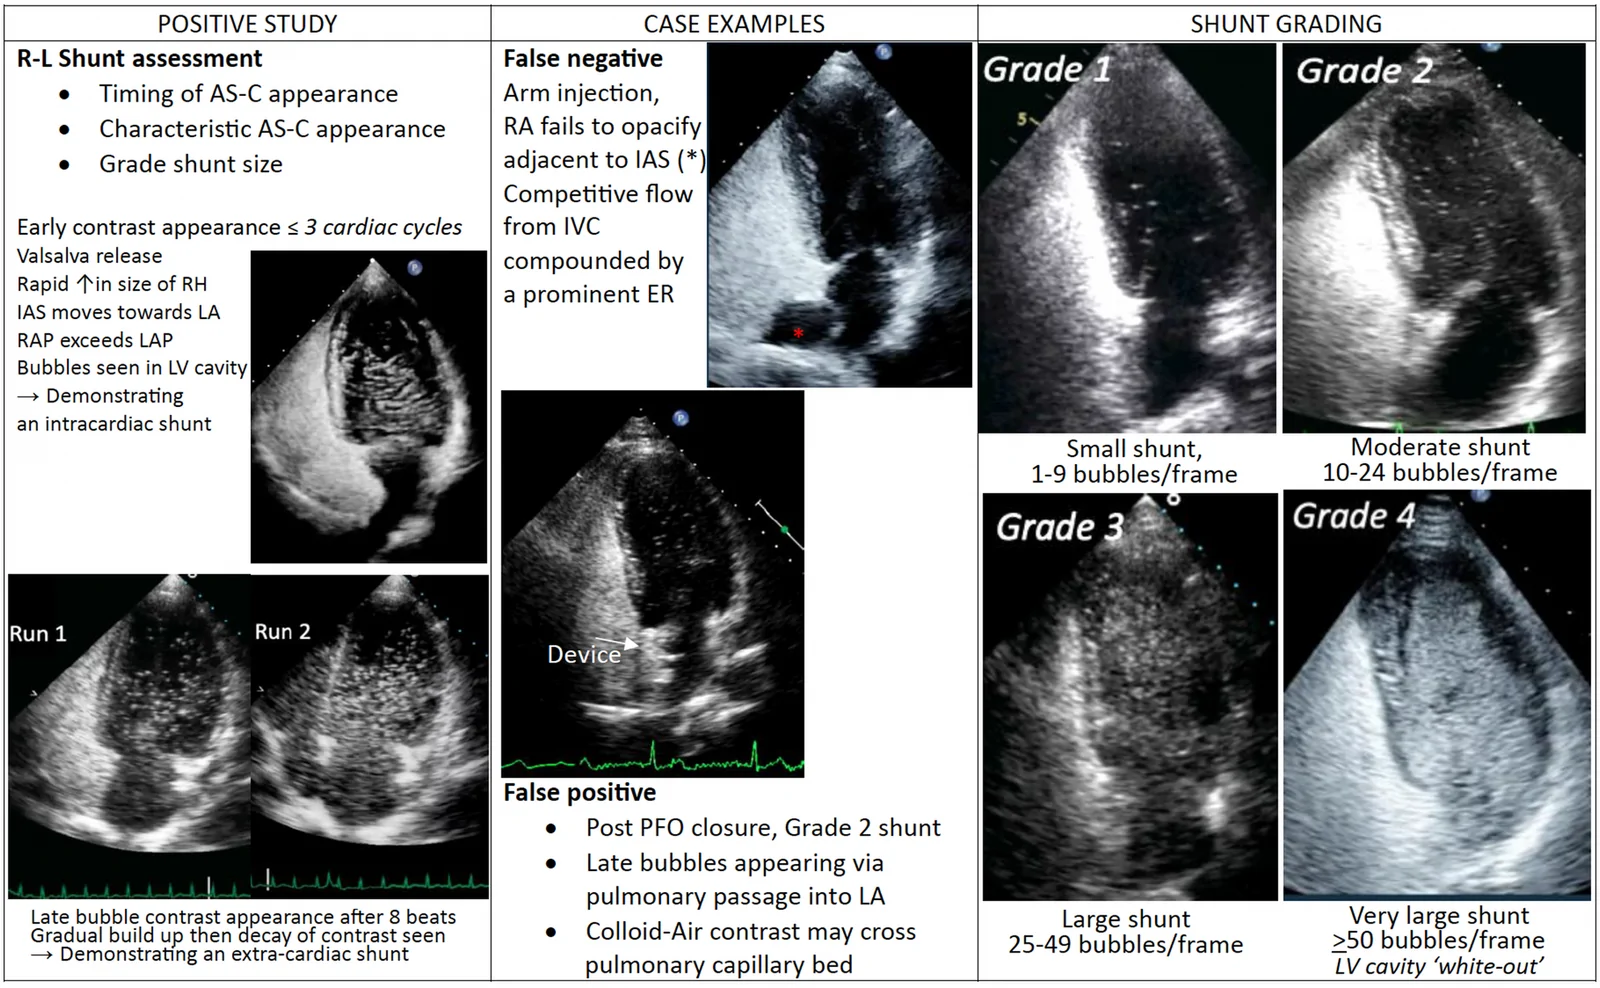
Agitated Saline Contrast Transthoracic echocardiography protocol. Diagnosis of a right to left shunt is described in the left panel. Case examples are depicted in the middle panel and describe commonly seen scenarios associated with false positive and false negative studies. The far right panel shows examples of shunt grades 1–4. *R-L right to left*,* LA left atrium*,* RA right atrium*,* RAP right atrial pressure*,* LAP left atrial pressure*,* RH right heart*,* IAS inter-atrial septum*,* ER Eustachian ridge*,* PFO patent foramen ovale*,* IVC inferior vena cava*
